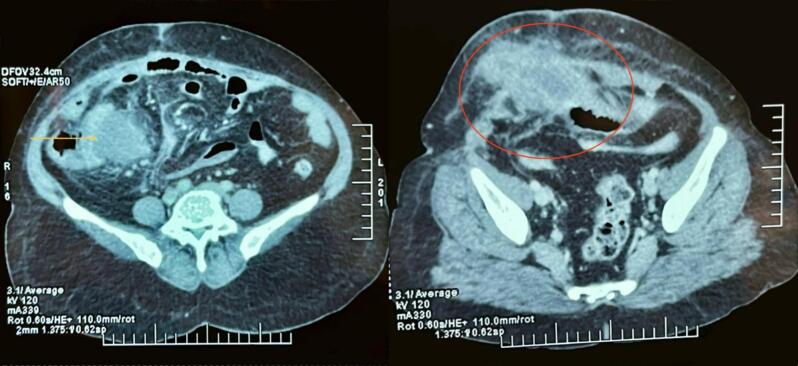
CECT of abdomen and pelvis axial view shows a peripherally enhancing lesion with a thick, irregular wall and a central collection in the right lower abdomen (yellow arrow), extending into the right anterior abdominal wall (red circle). CECT: Contrast-Enhanced Computed Tomography. (For interpretation of the references to colour in this figure legend, the reader is referred to the web version of this article.)
